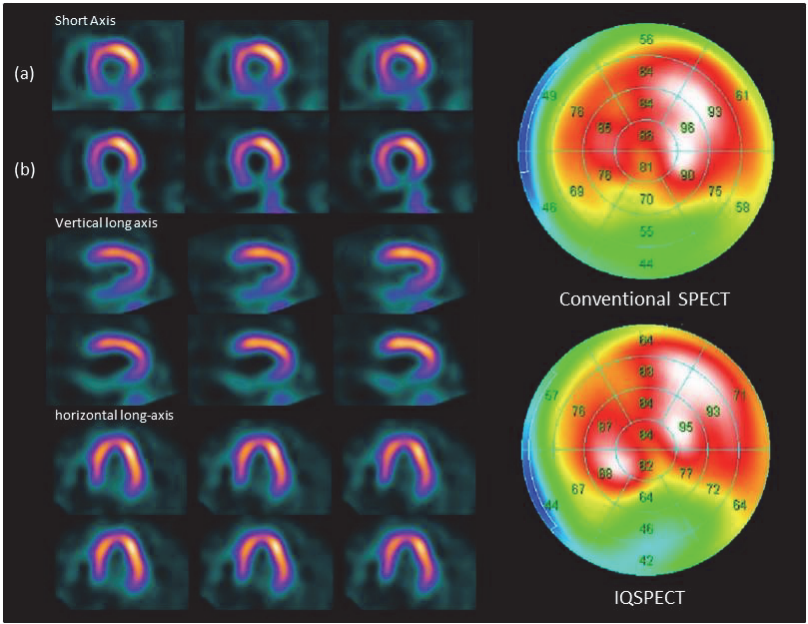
^99m^Tc-tetrofosmin MPI SPECT of a 74-year-old male with an old myocardial infarction in the inferobasal wall. Both IQ-SPECT and conventional SPECT show perfusion defect in the inferobasal wall; (a) conventional SPECT, (b) IQ-SPECT
